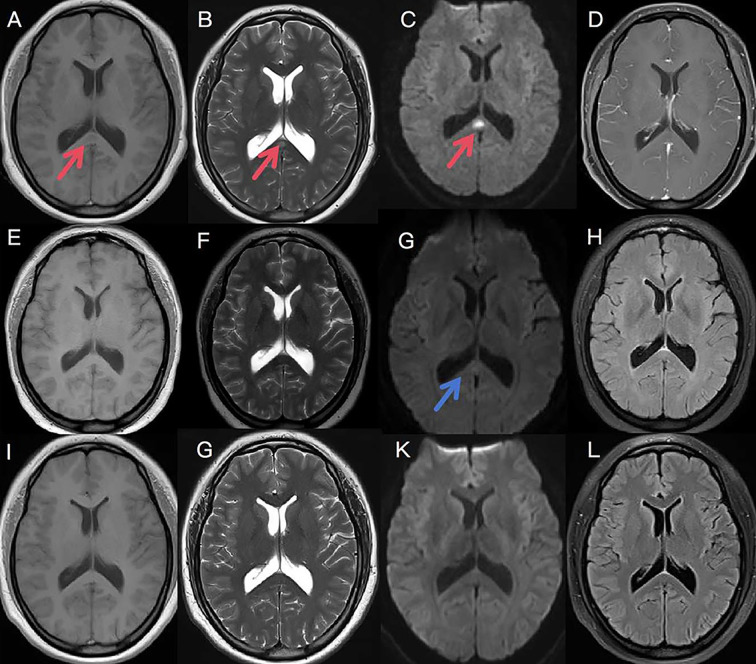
Representative MRI images of the splenium of the corpus callosum in a patient with GFAP-A. (A-D) Initial presentation at admission. Red arrows indicated lesions in the splenium of the corpus callosum.Brain MRI showed hypointensity on the T1-weighted image (A), hyperintensity on T2-weighted (B) and diffusion-weighted imaging (DWI) MRI (C), but no enhancement on the post-contrast T1-weighted image (D). (E-H) Follow-up images approximately 2 weeks after initiation of mmunotherapy. Near-complete resolution of the lesion was observed on T1 (E), T2 (F), and post-contrast T1 (H) sequences.A decreased signal intensity is noted on DWI (G) (blue arrow). (I-L) Follow-up images at about 5 months after discharge, demonstrating complete resolution of the lesion.
